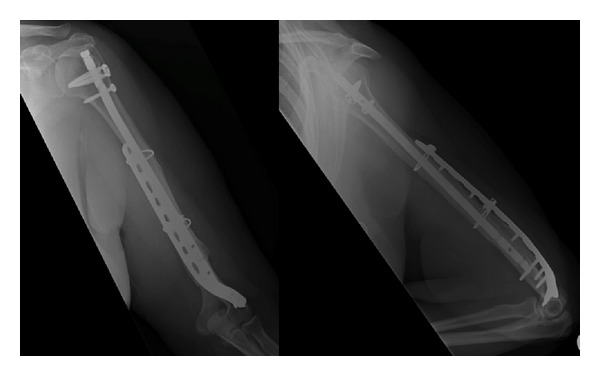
AP and lateral radiographs demonstrating union of distal humeral periprosthetic fracture.
